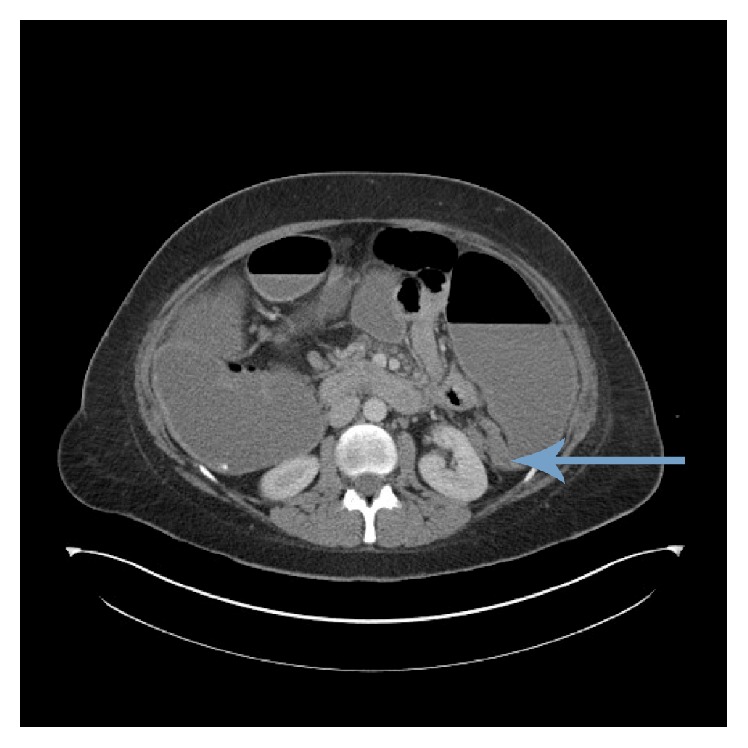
CT scan demonstrating large bowel obstruction with arrows showing cut-off at splenic flexure.
